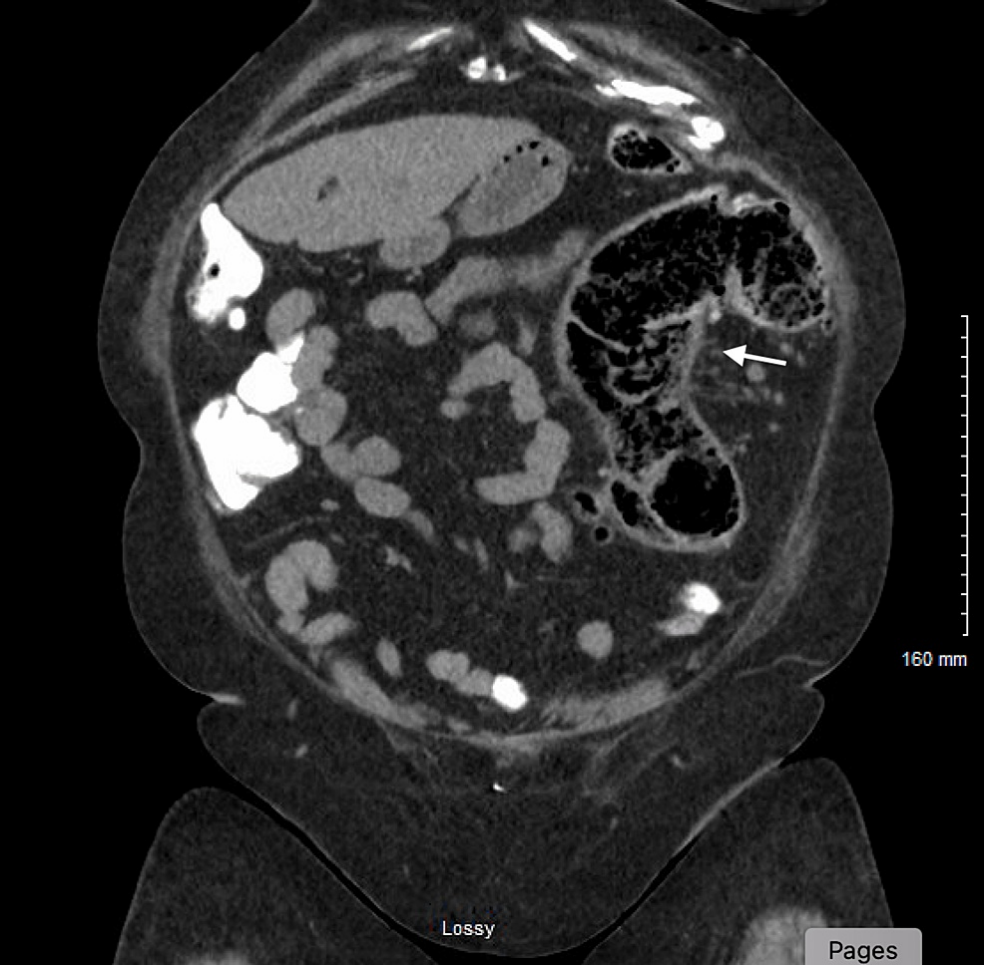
CT abdomen showing abnormal inflammatory changes suggestive of diverticulitis in the colon. CT: computed tomography
